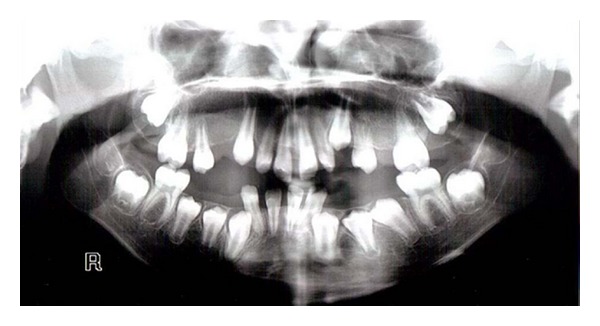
Panoramic radiograph showing normal development of permanent teeth and missing of maxillary right first and left second premolars.
